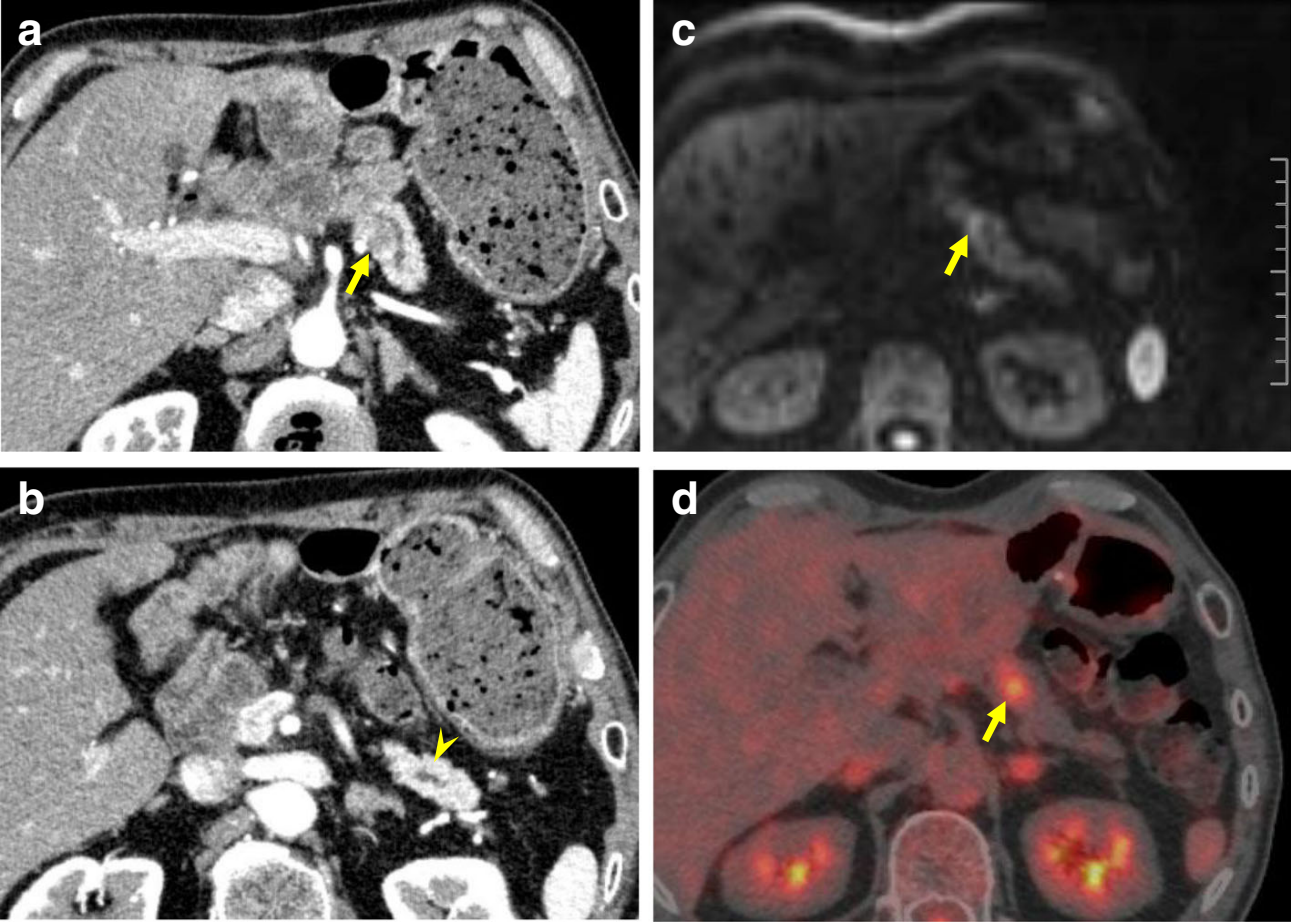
Preoperative image findings in the second surgery. a CT showed a low concentration region of 2 cm in size near the pancreato-jejunal anastomosis (arrow). b Dilatation of the upstream main pancreatic duct was observed (arrowhead). c MRI showed a tumor with a high signal by DWI at the stenosis of the pancreatic duct. d PET-CT revealed the accumulation of SUVmax 4.0 at the tumor
